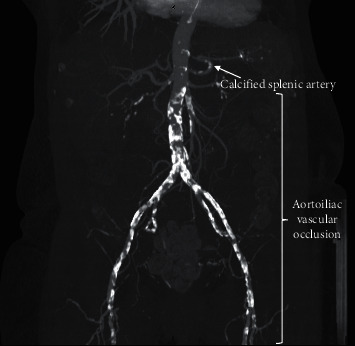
A CT reconstruction showing aortoiliac vascular occlusion and calcified splenic artery. This is a complex case that could require the anastomoses to the native renal vessels.
